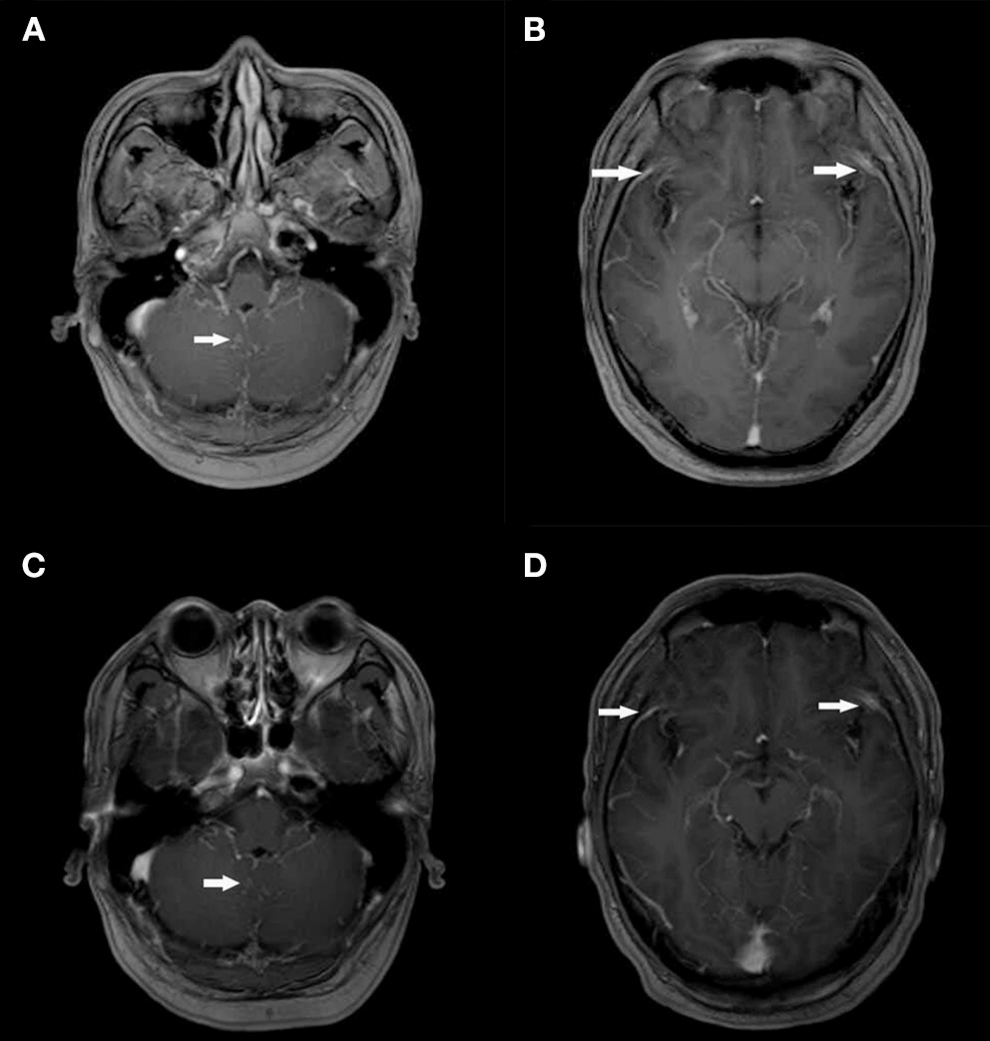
Contrast-enhanced and Diffusion-weighted Cranial MRI Scans on Day 3 of Admission and after 6 Months. On the third day of admission, post-contrast T1 MRI showed partial cerebellar surface enhancement (A,B) and get better at 6 months later after discharge (C,D).
